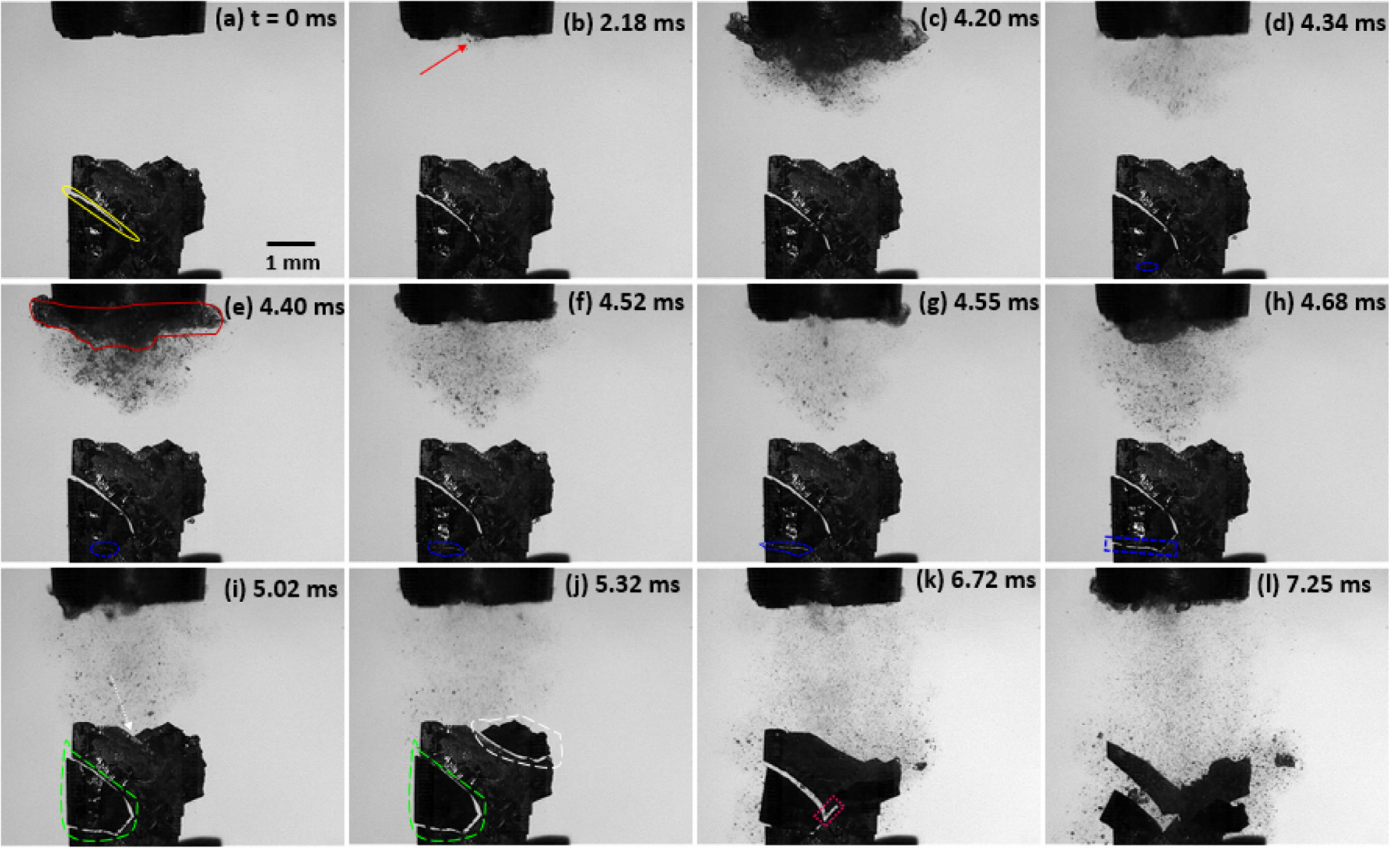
High-speed images of fragmentation of primary Al_3_Zr (single edged notch) crystals captured at 100 kfps when subjected to ultrasonic cavitation. The supplementary video can be accessed with the online version of the article as video 3.
